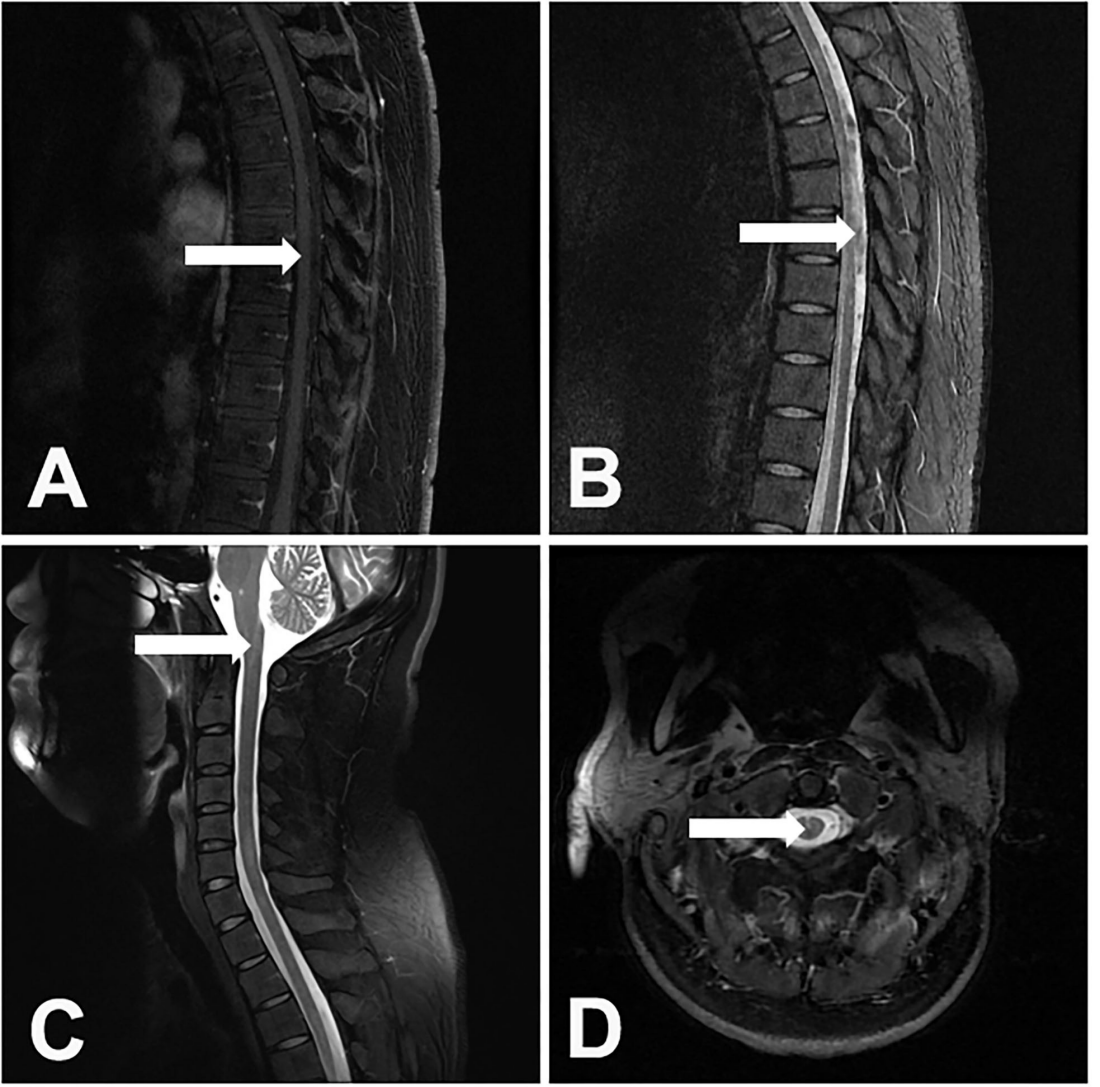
A 31-year-old male patient developed paradoxical myelitis. The patient developed new spinal cord lesions 3 months after the start of antituberculosis treatment, when his symptoms of headache, fever, and disturbance of consciousness were gradually alleviated. A T1-weighted sagittal image of the thoracic spinal cord of the patient showed a spot-like hypointensity (A). In the T2-weighted sequence, multiple patchy hyperintensities in the spinal cord can be seen (B). T2-weighted sagittal and axial images of the cervical spinal cord showed an enlargement of the central canal in the spinal cord (C,D).
